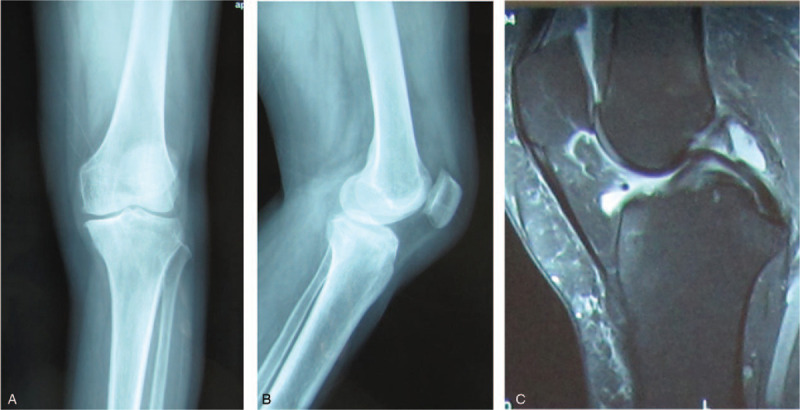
a, b: Anteroposterior and lateral views of the left knee before UKA, showing localized medial unicompartmental osteoarthritis. c:MRI showing intact ACL and PCL.
